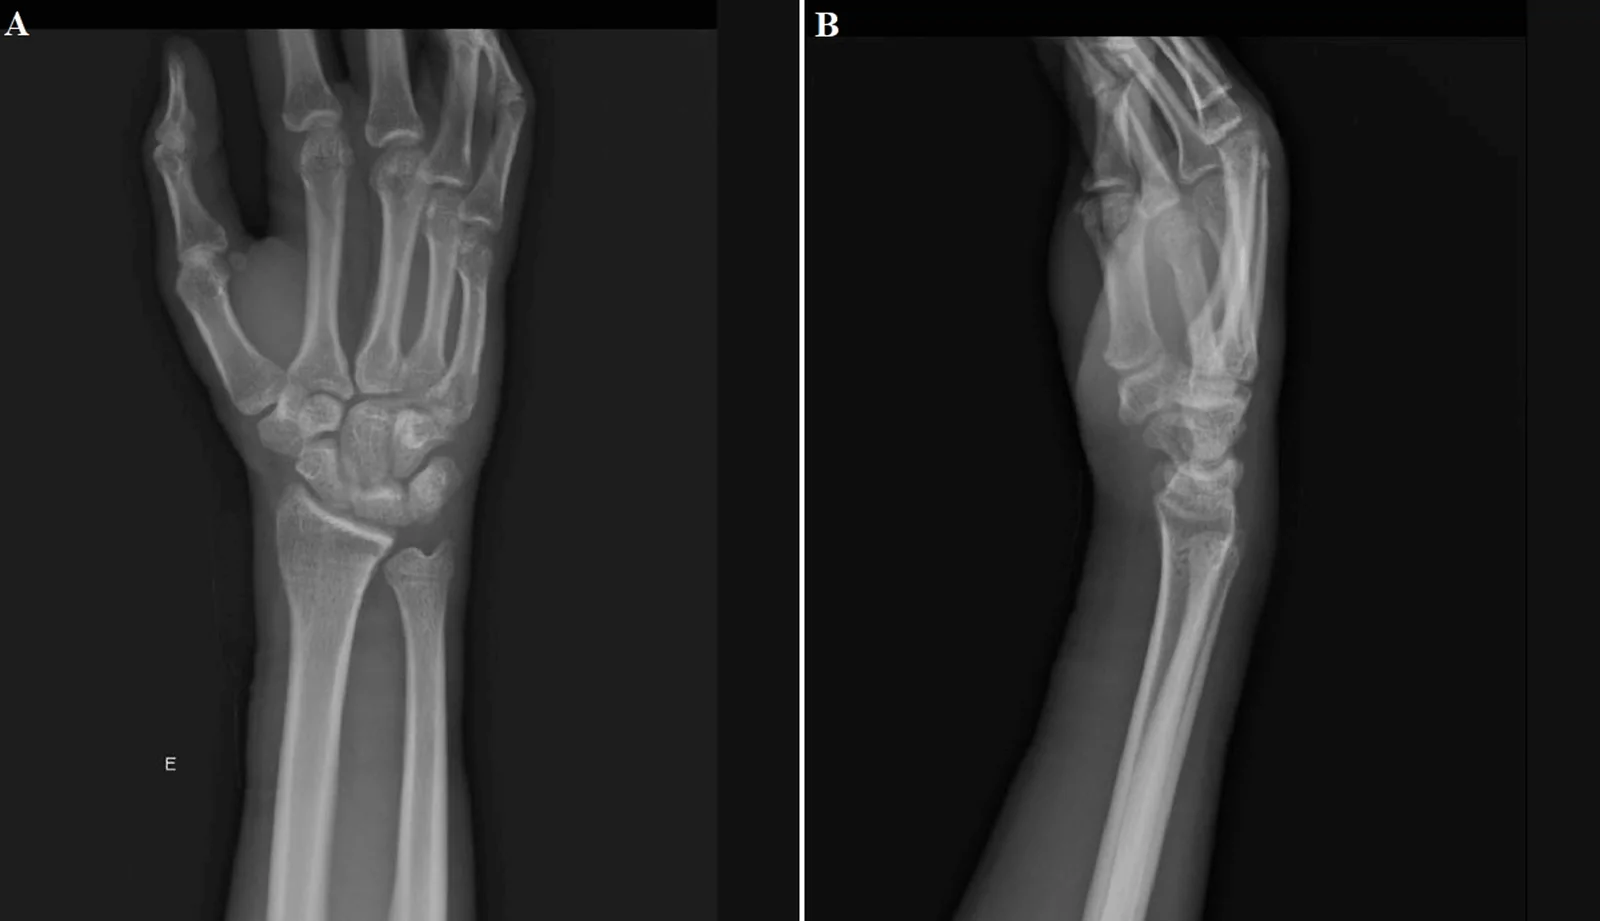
Radiographs four weeks after surgery following removal of the Kirschner wires and plaster splint. (A) Anteroposterior view. (B) Lateral view showing maintained alignment.
